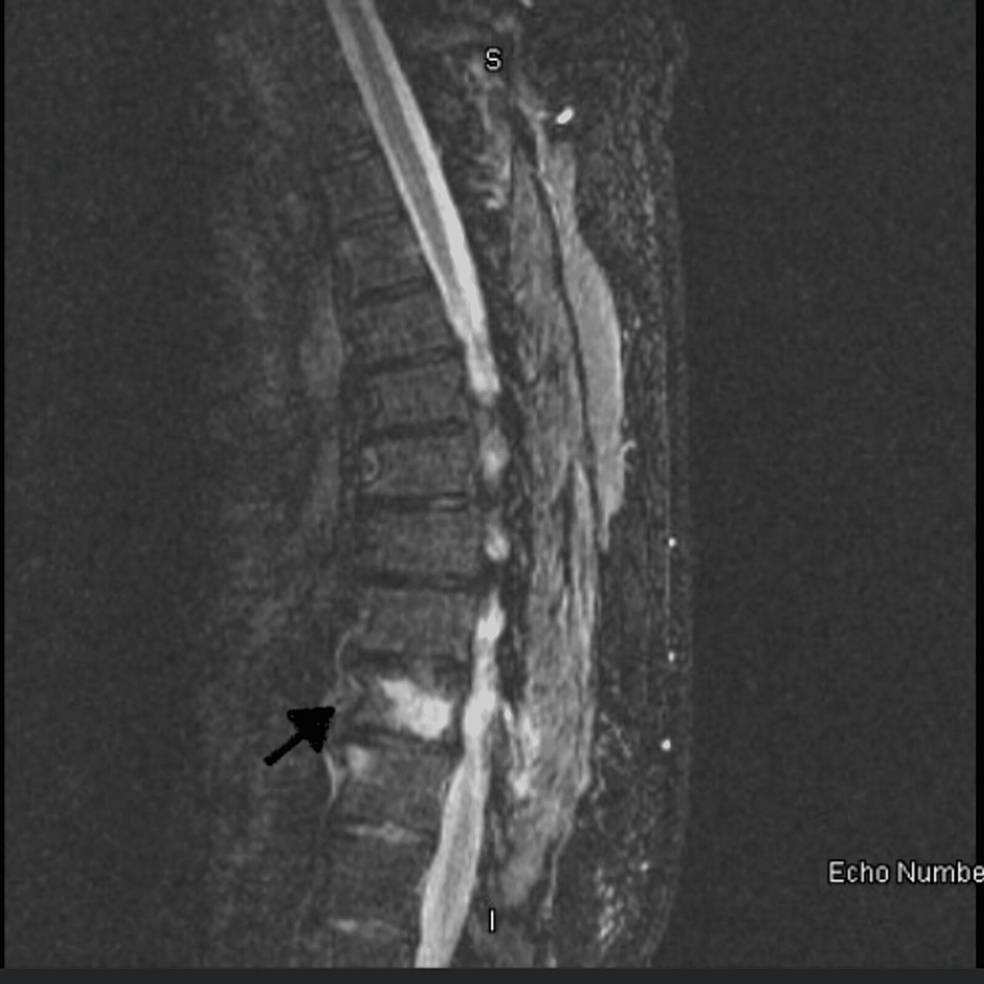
MRI of the spine with T10 vertebral body enhancement with abnormal signal focus, labelled with the black arrow (sagittal view)
